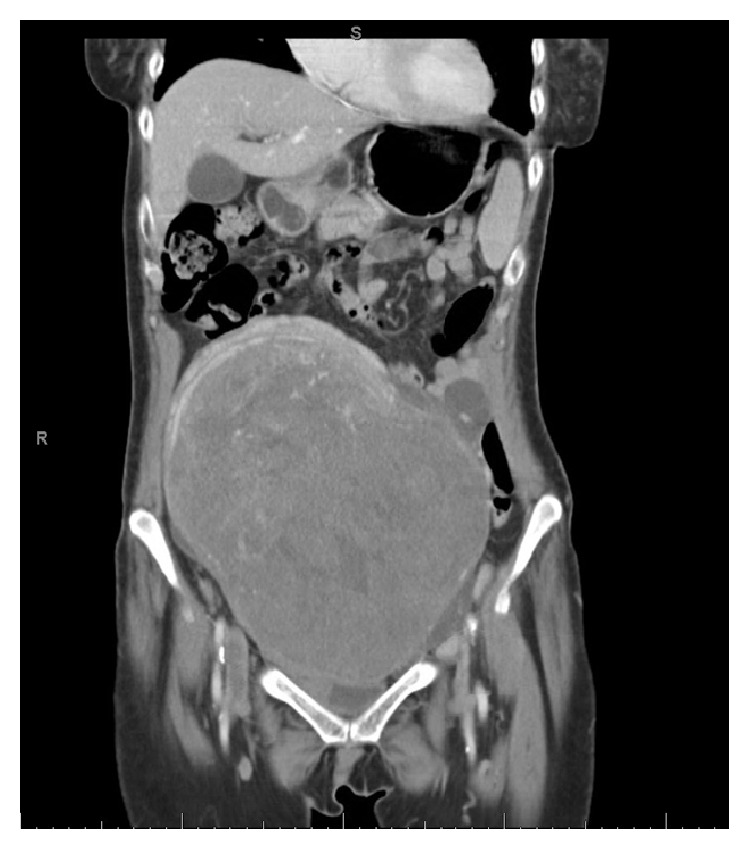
Abdomen-pelvis CT scan of case 2 showing large uterine leiomyoma.
